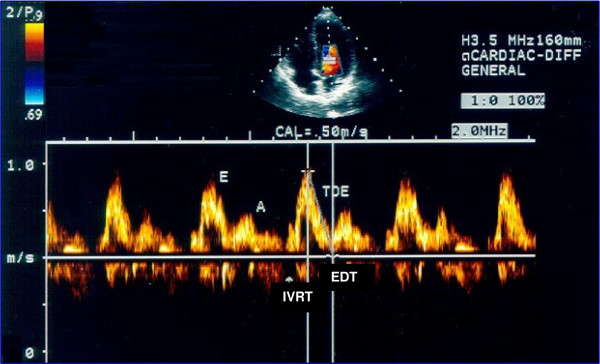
LV diastolic indices measured by transmitral Doppler. E: LV early filling flow velocity; A: late filling flow velocity; EDT: E-wave deceleration time; IVRT: LV isovolumic myocardial relaxation time.
